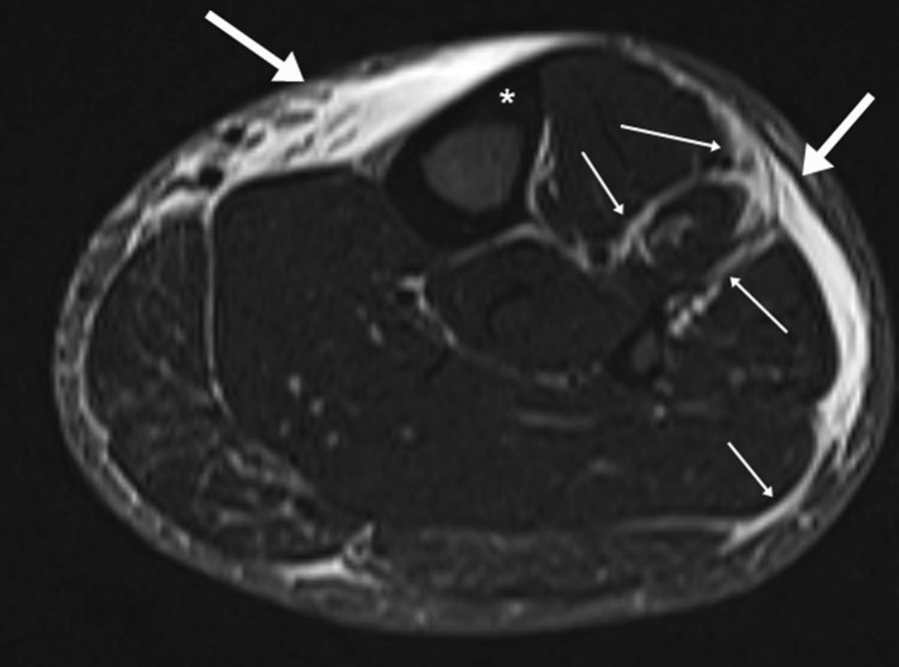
High water-sensitive MRI of the left lower leg (TIRM: turbo inversion recovery magnitude): severe “shin-splint” leading to premature termination of TEFR (47 years, male, stage 5 of TEFR, after 261-km run). *Thick arrow*: panniculitis, epifasciitis; *thin arrow*: myofasciitis and intermuscular fasciitis (extensors of the lower leg); * inert cortical bone (Tibia) without any periosteal bone reaction
